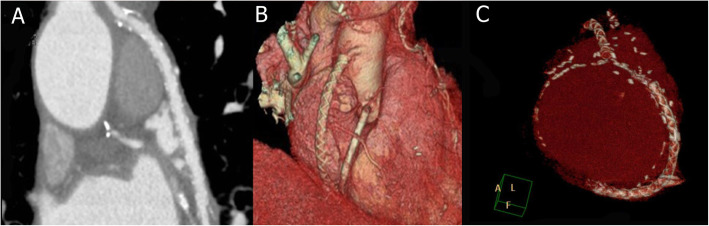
CT angiography of externally stented SVG to the right coronary artery in a 2D (a) and 3D reconstruction (b), and a 3D reconstruction of externally stented sequential SVG to the ramus intermediate and the posterior descending arteries (c).
